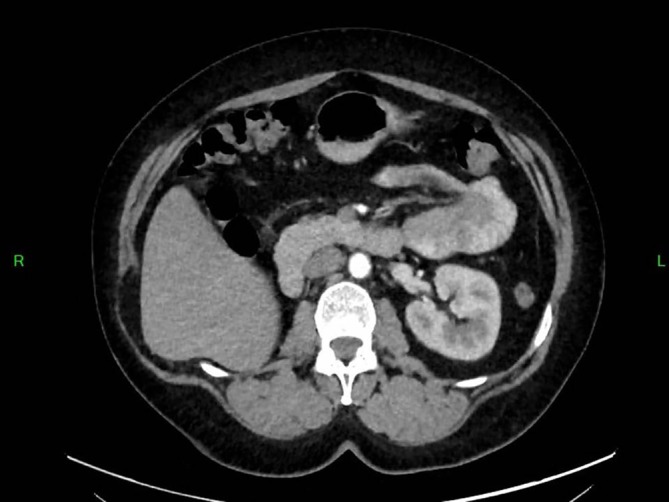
Follow‐up abdominal CT scan obtained 19 months after right nephrectomy, showing no abnormal findings and no radiological evidence of recurrent or residual abdominal disease.
